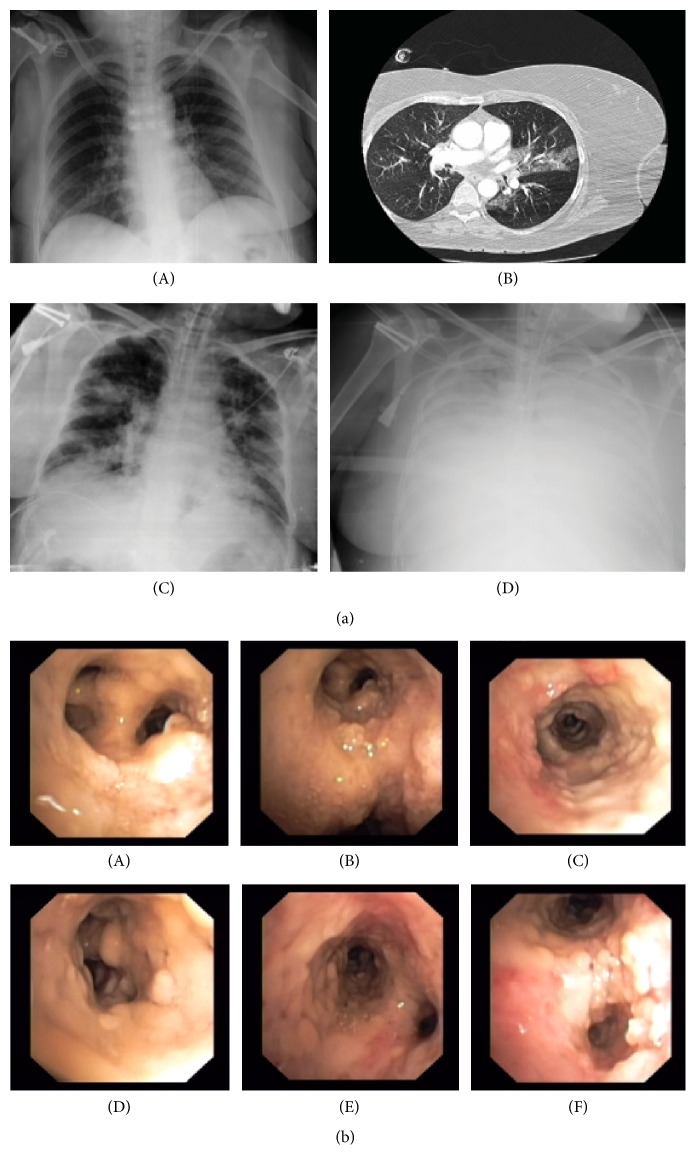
Radiological and bronchoscopic findings. (a)* Radiological findings.* (A) Chest radiography (CRx) at hospital admission (day 1). (B) Computed tomography revealing small lingula consolidation (day 2). (C) CRx at ICU admission with new bilateral infiltrates (day 3). (D) CRx after 24 h of ICU admittance showing bilateral atelectasis (day 4). (b)* Bronchoscopic findings.* (A) Necrotic detritus and pseudomembrane in the right upper lobe. (B) Right upper lobe and right carina with an extensive deposit of necrotic material. Bronchoscopic biopsy was performed and revealed the presence of fungus with hyphae suggestive of* Aspergillus*. (C) Involvement of intermediate bronchus. (D) The orifice of bronchus in the culmen was not visible because of a large quantity of necrotic material. Bronchial wash fluid revealed the presence of* Aspergillus*. (E) Necrotic detritus and pseudomembrane in the left upper lobe. (F) Involvement of culmen, left carina, and lingula.
